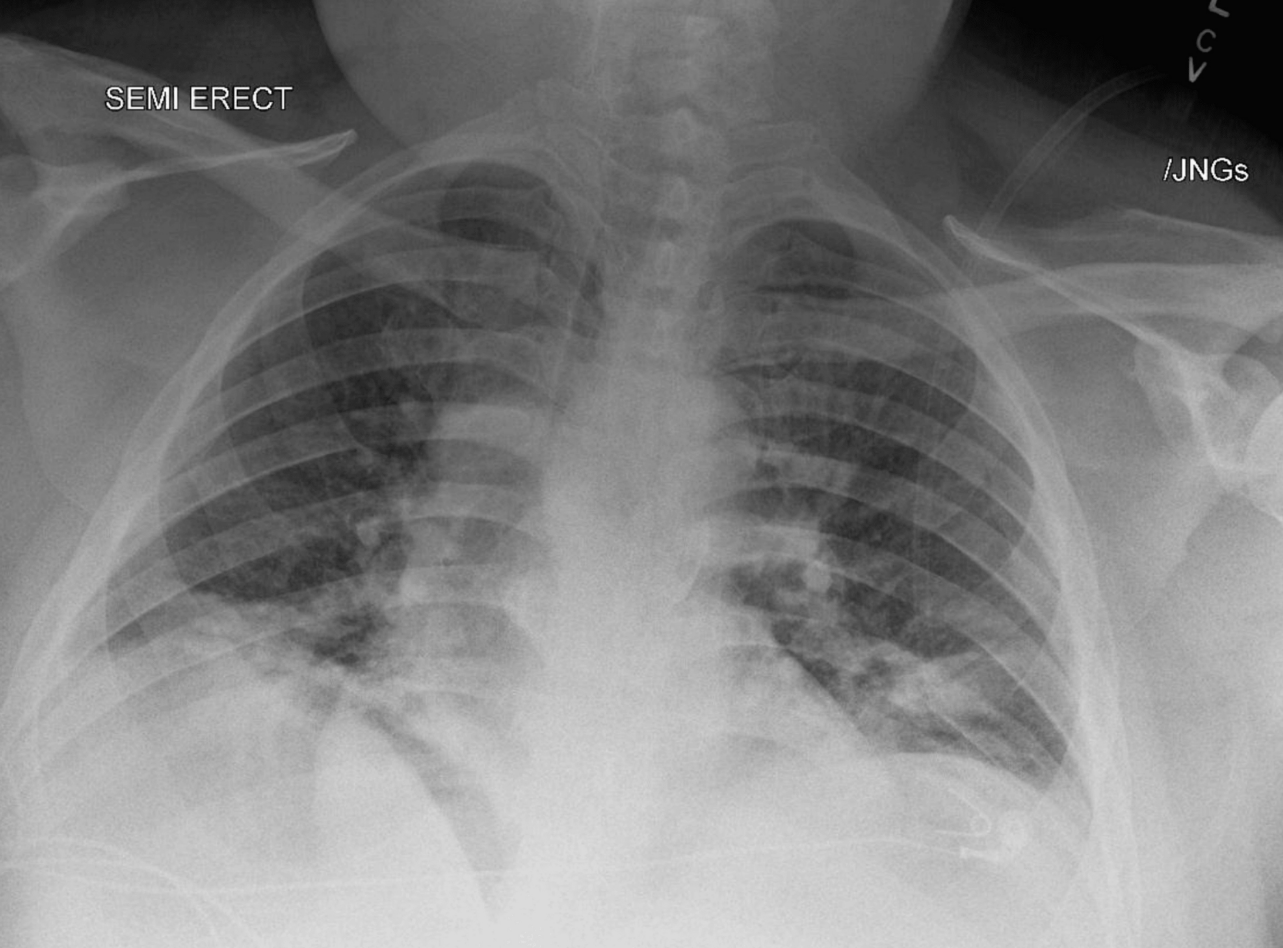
Chest X-ray demonstrating bibasilar atelectasis and mild fluid overload causing pulmonary edema.
